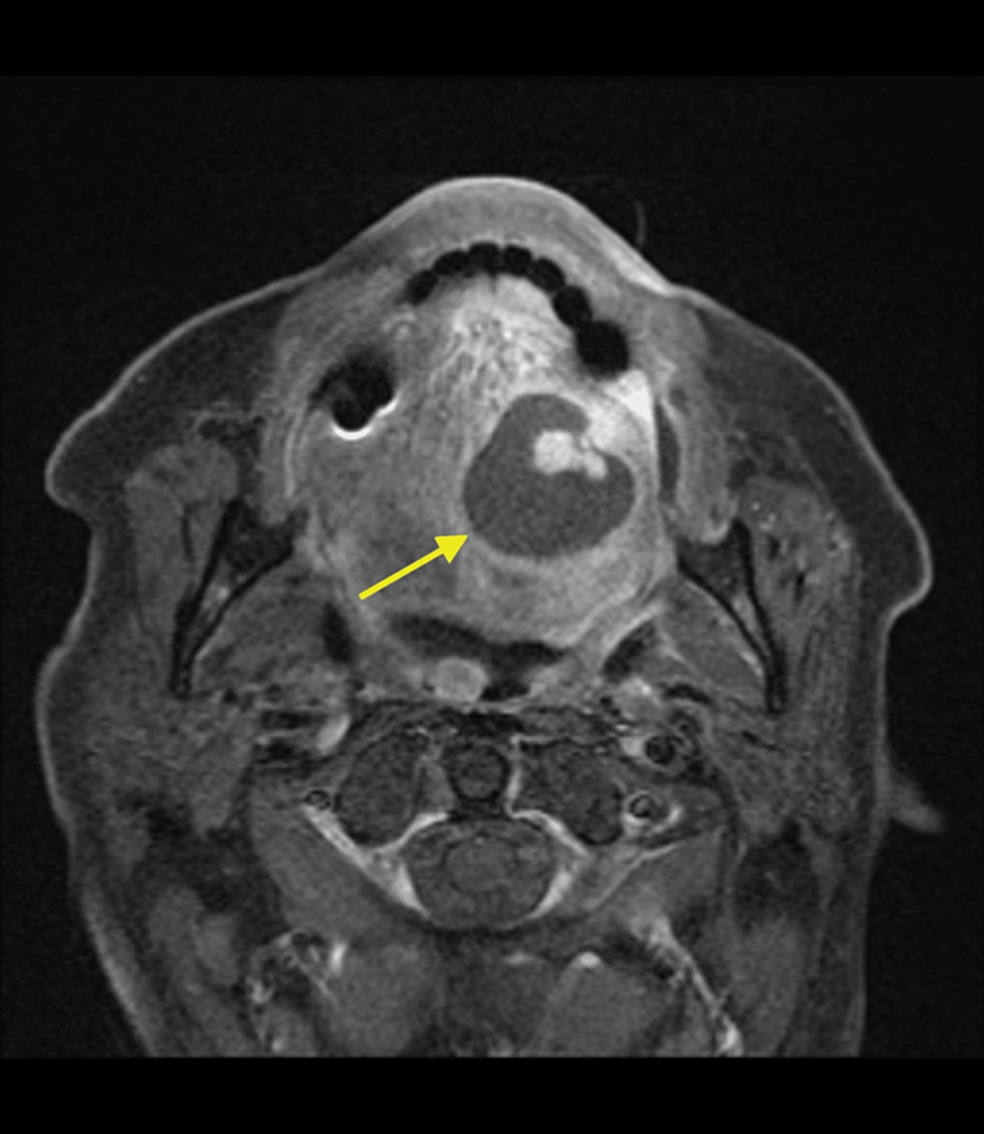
Axial postcontrast fat-suppressed T1-weighted magnetic resonance image of the neck showing avid 1.2 cm central nodular enhancement within the lesion with surrounding fluid hypoenhancement (arrow), most consistent with a lingual artery pseudoaneurysm with surrounding hematoma.
